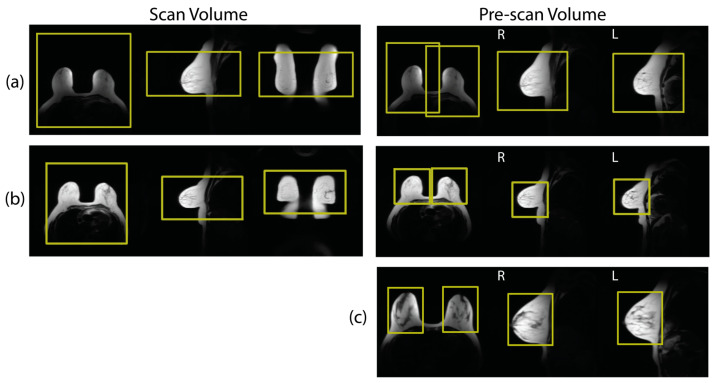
Examples (a,b) show scan volumes and pre-scan volumes as prescribed during clinical breast MRI. Both (a,b) come from the same patient but from exams on different days. Note that the placement of scan volumes was relatively consistent across both exams. However, the pre-scan volume placements were dramatically different between exams. Example (c) shows an example from a different patient with offline volumes generated by expert users that was used to train pre-scan volume placement.
